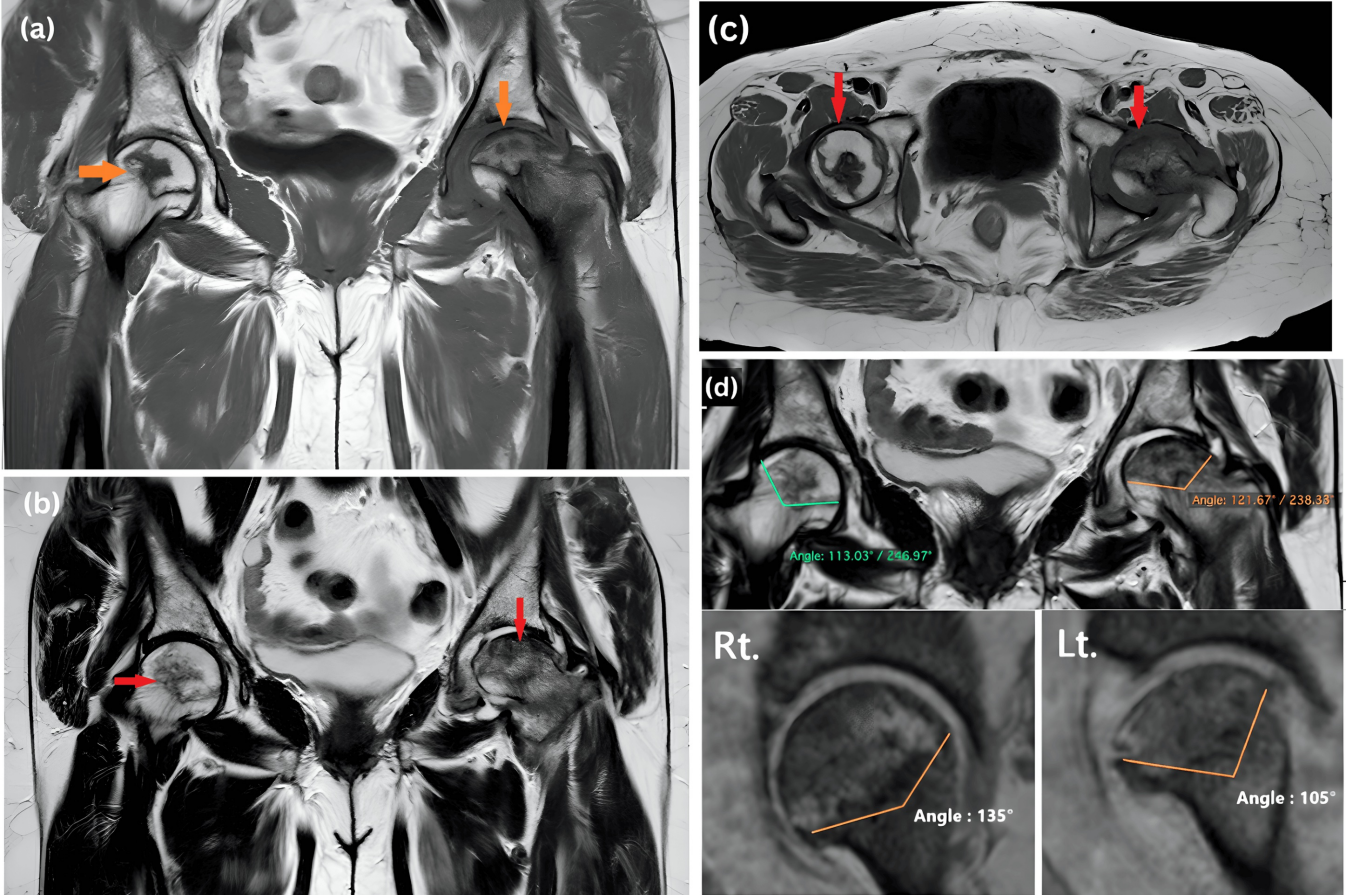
(a) T1W coronal image shows a focal geographic area with a double line sign and subchondral cyst noted on the left side. Significant joint space narrowing is noted. Minimal cortical irregularities are noted in the right femoral head with mild narrowing of the joint space (arrows). (b) T2W coronal image shows bone marrow edema on the left side with mild joint effusion (arrows). (c) T1W axial image shows a geographic area of mixed signal intensity bilaterally, predominantly in the antero-latero-superior quadrant. However, no femoral head collapse was noted (arrows). (d) Modified Kerboul's angle right: -248°(113°+135°); modified Kerboul's angle left: -232° (127°+105°). Findings suggestive of bilateral AVN of the hip joint consistent with ARCO stage II on the right side and ARCO stage IIIA on the left side. T1W: T1-weighted, T2W: T2-weighted, °: degree, ARCO: Association Research Circulation Osseous classification
